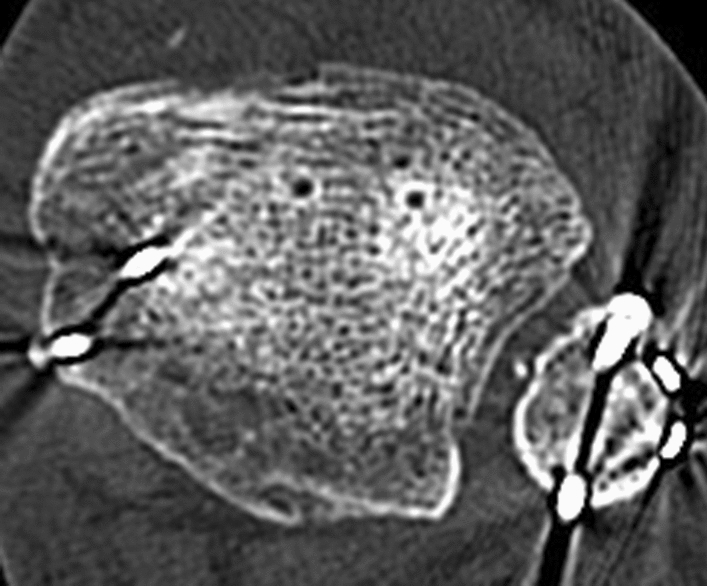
Postoperative CT control. a axial scan; b frontal scan
